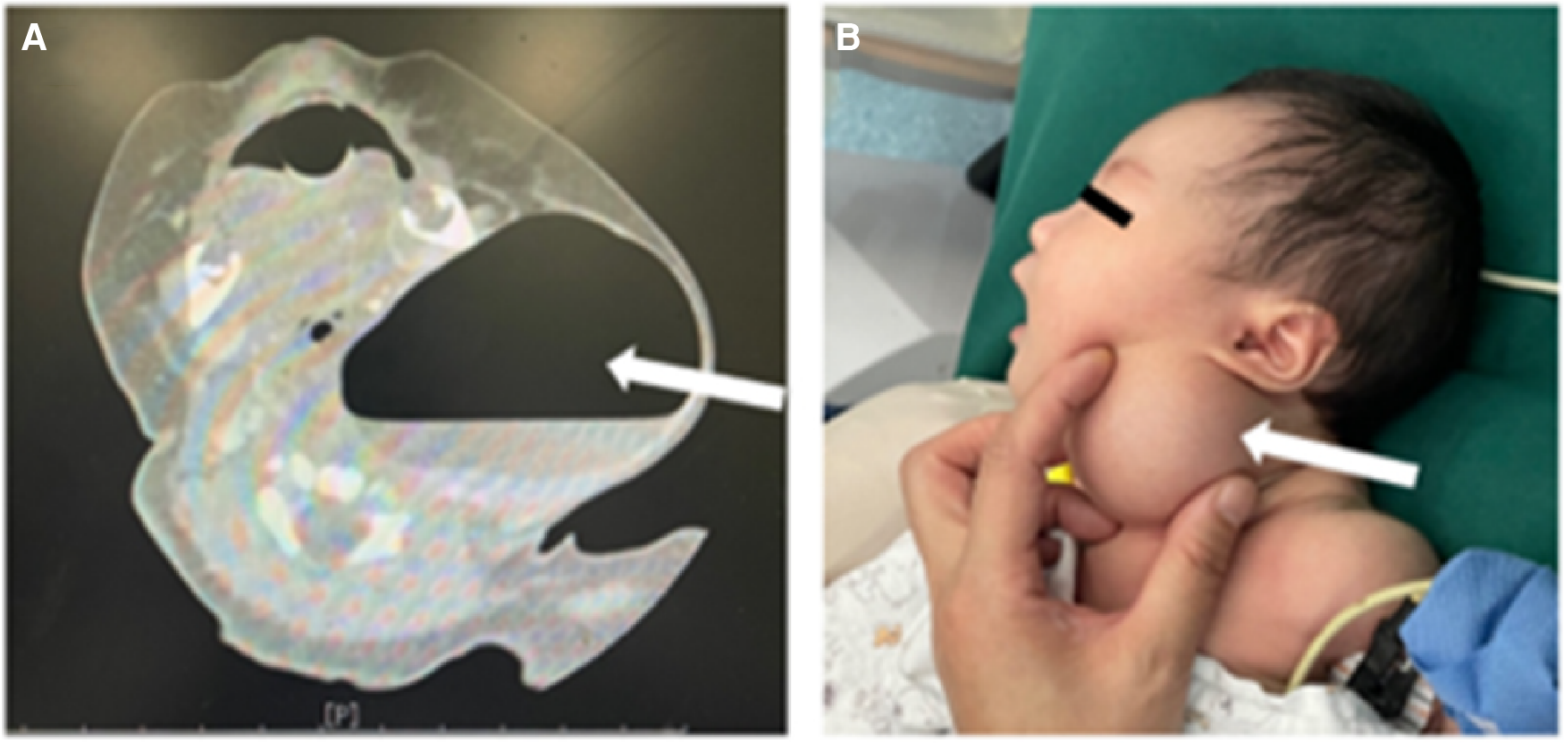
Giant piriform fossa cyst of newborn. (A) CT images; (B) clinical photograph. White arrow: giant piriform fossa cyst.
